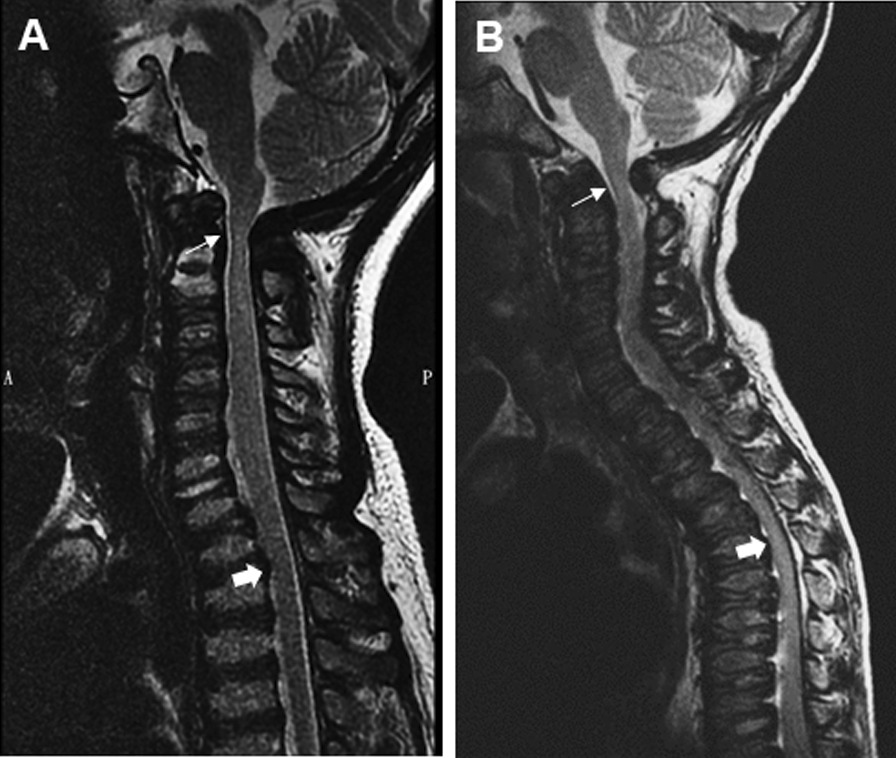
Spinal MRI images demonstration in MPS IVa patients. A MPS type IVa in a 14-year-old girl (subtype 1). Sagittal T2-weighted FSE MRI of the spine shows spinal canal narrowing around bony level of T2 (solid arrow) corresponding to CT manifestation of cervicothoracic kyphosis in addition to common spinal stenosis site around cervicomedullary junction (arrow). B MPS type IVa in a 5-year-old girl (subtype 2). T2-weighted FSE MRI of the spine in sagittal plane shows narrowing of the spinal canal more significant around bony level of T5 (solid arrow) related to CT reveals middle thoracic kyphosis. Atlantoaxial instability with mild cord myelopathy around cervicomedullary junction is also evident (arrow)
